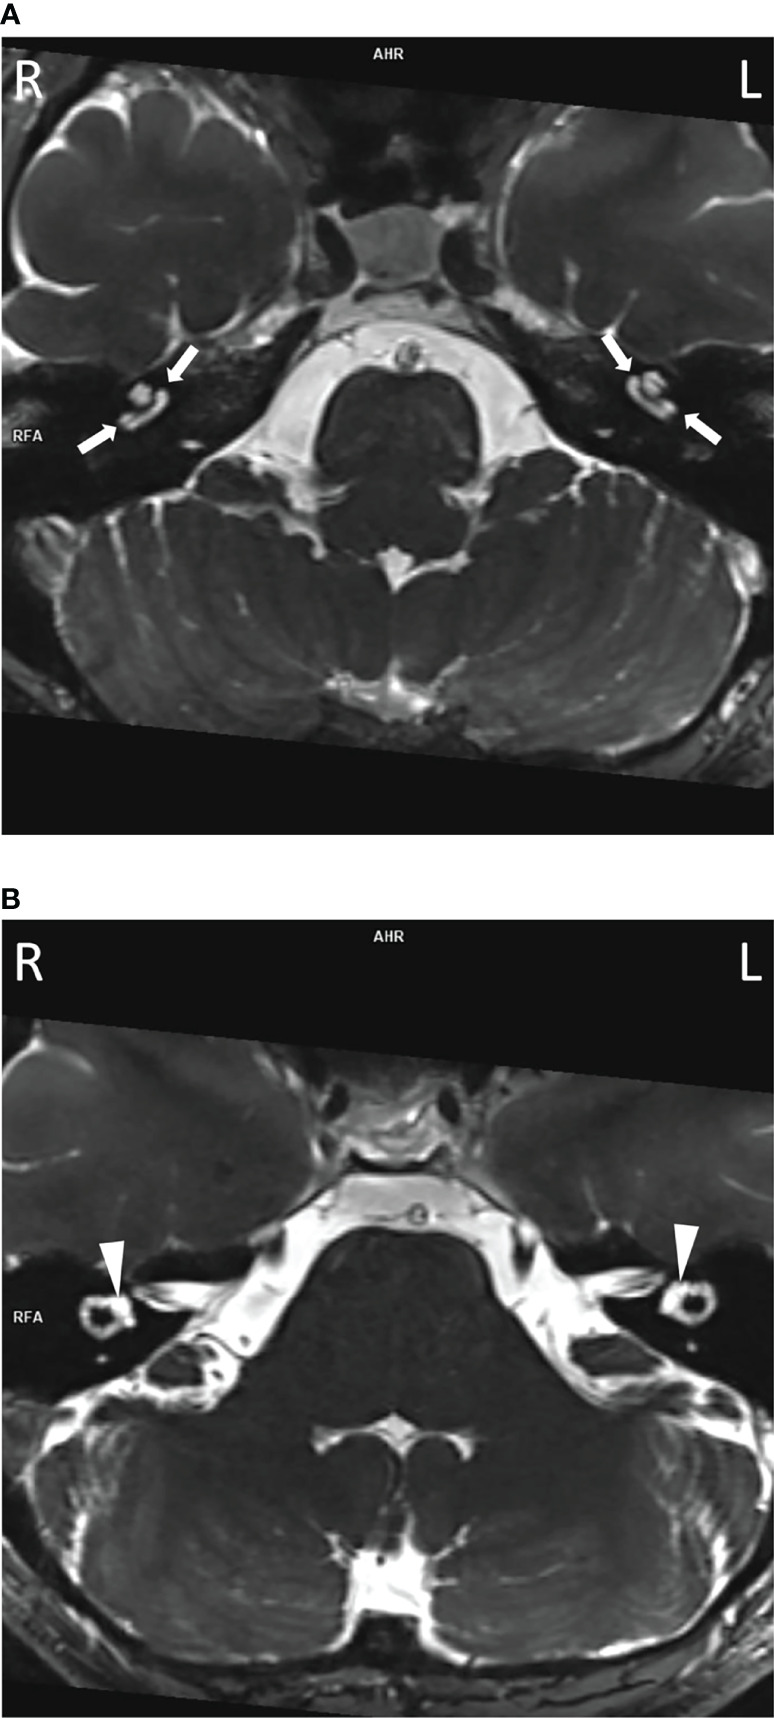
The decreased bright cochlear fluid signal on T2-weighted images in the proband. Slight diminishing of the bright fluid signal was visible in both cochleas (A, arrows) compared to vestibules (B, arrowheads) and more pronounced on the left.
